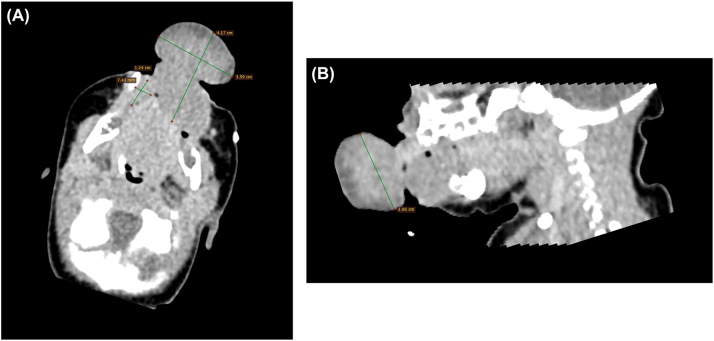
CT images of the lesion: (A) Axial CT image showing the lesion, total length 41 mm, extraoral component 35 mm in diameter, with a smaller similar lesion measuring 12 × 7 mm. (B) Sagittal CT image demonstrating the lesion, vertical extent measured 30 mm.
